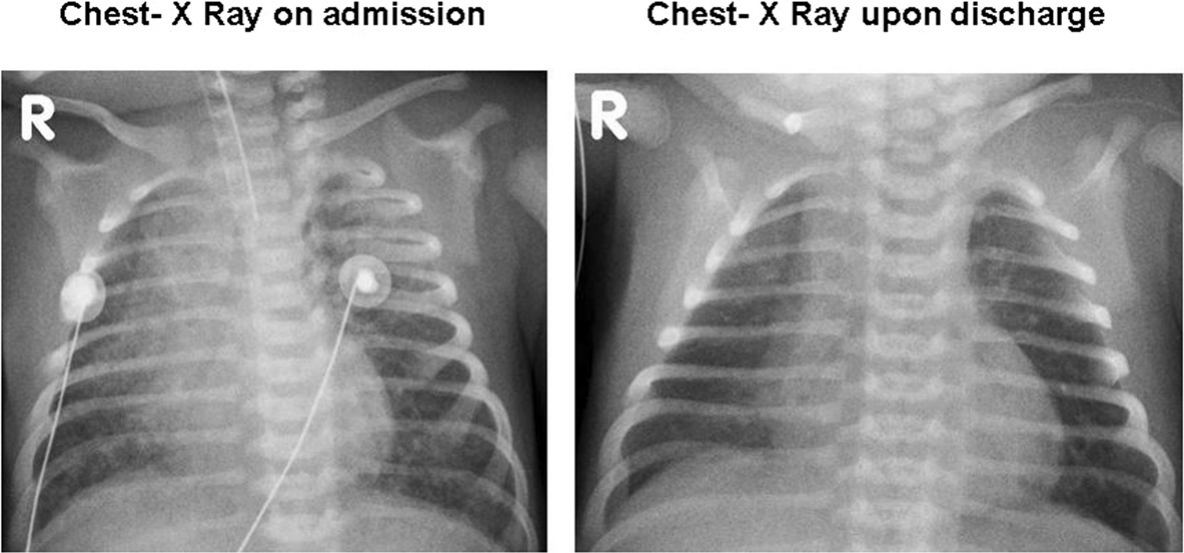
Chest X-rays showing bilateral patchy infiltrates due to pulmonary hemorrhage on admission and resolution upon discharge.
